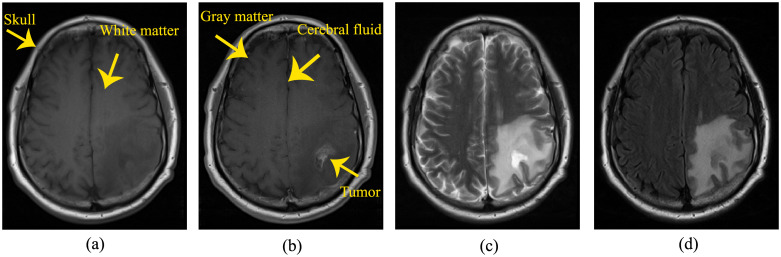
Four MR images of a glioblastoma patient with an irregular lesion in the parietal lobe of the left hemisphere. (a) T1 weighted, (b) T1 weighted post-contrast agent, (c) T2 weighted, and (d) T2 weighted FLAIR.
